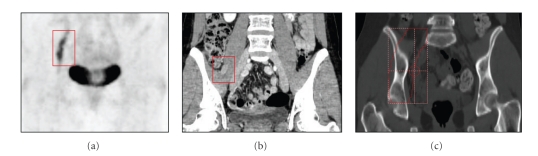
Recurrent neurotropic chloroma of the right femoral nerve outlined in red on (a) pretreatment Positron Emission Tomography (PET) and (b) coronal Computerized Tomography (CT) imaging with the (c) CT-based treatment field outlined in red.
